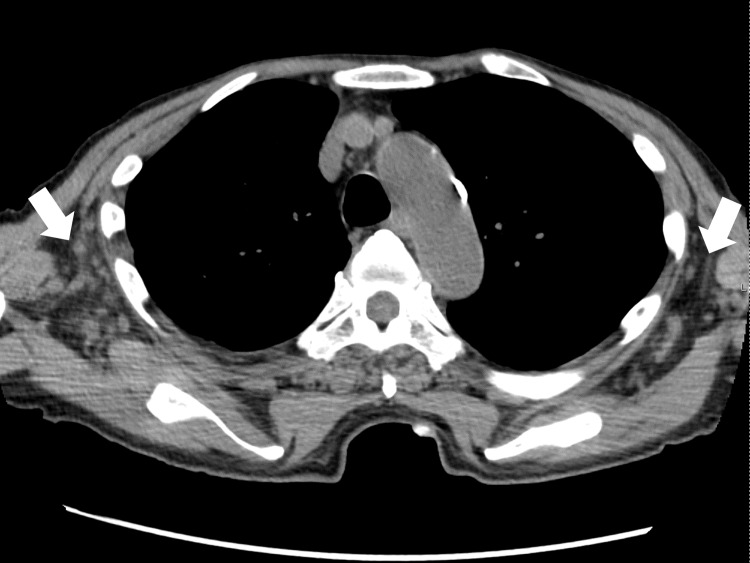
Computed tomography image of the chest showing lesions of axillary lymphadenopathy (white arrows).
